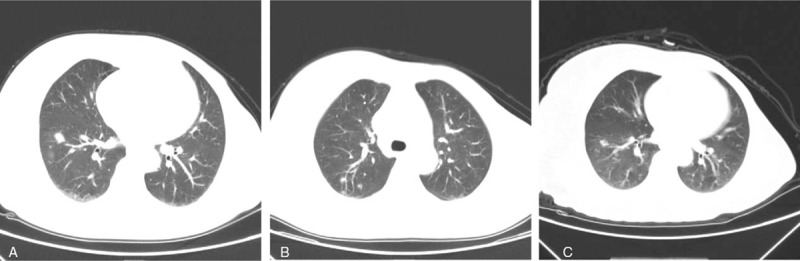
Findings of a chest CT. (A,B): The largest lesion was 14 mm in diameter. C: Findings of a chest CT after discontinuing the administration of anastrozole for 2 months.
